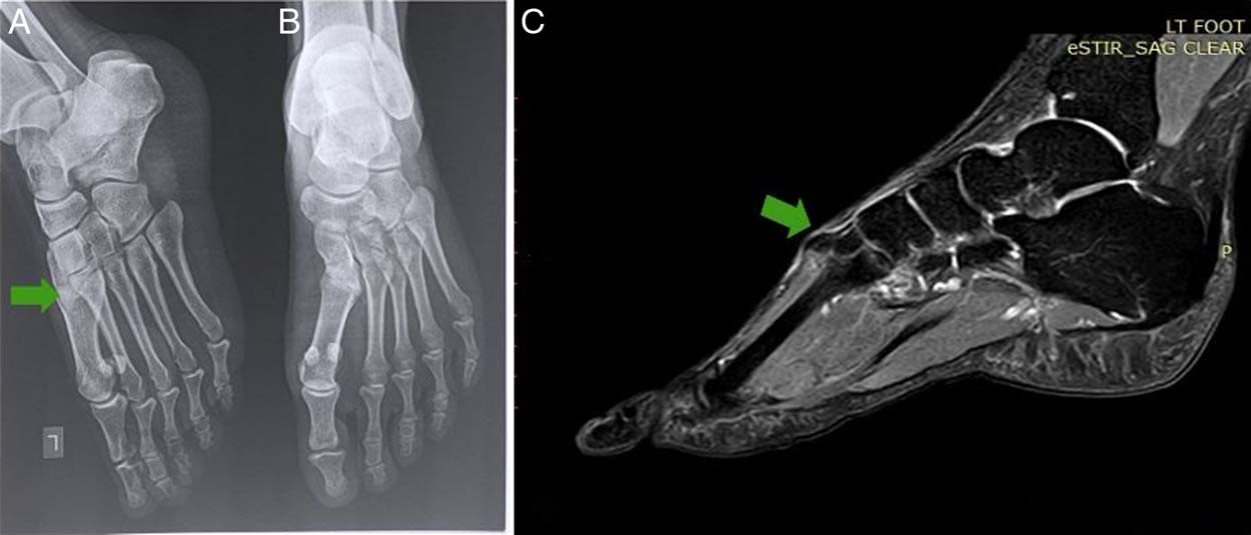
Radiograph of the left foot in oblique view (A) and anterior–posterior view (B) showing a radio-opaque lesion arising from the medial cuneiform. STIR MR sagittal section of left foot (C) showing a well-defined T1/T2 hyperintense and STIR hypointense bony outgrowth arising from the left medial cuneiform, with a thin cartilaginous cap.
